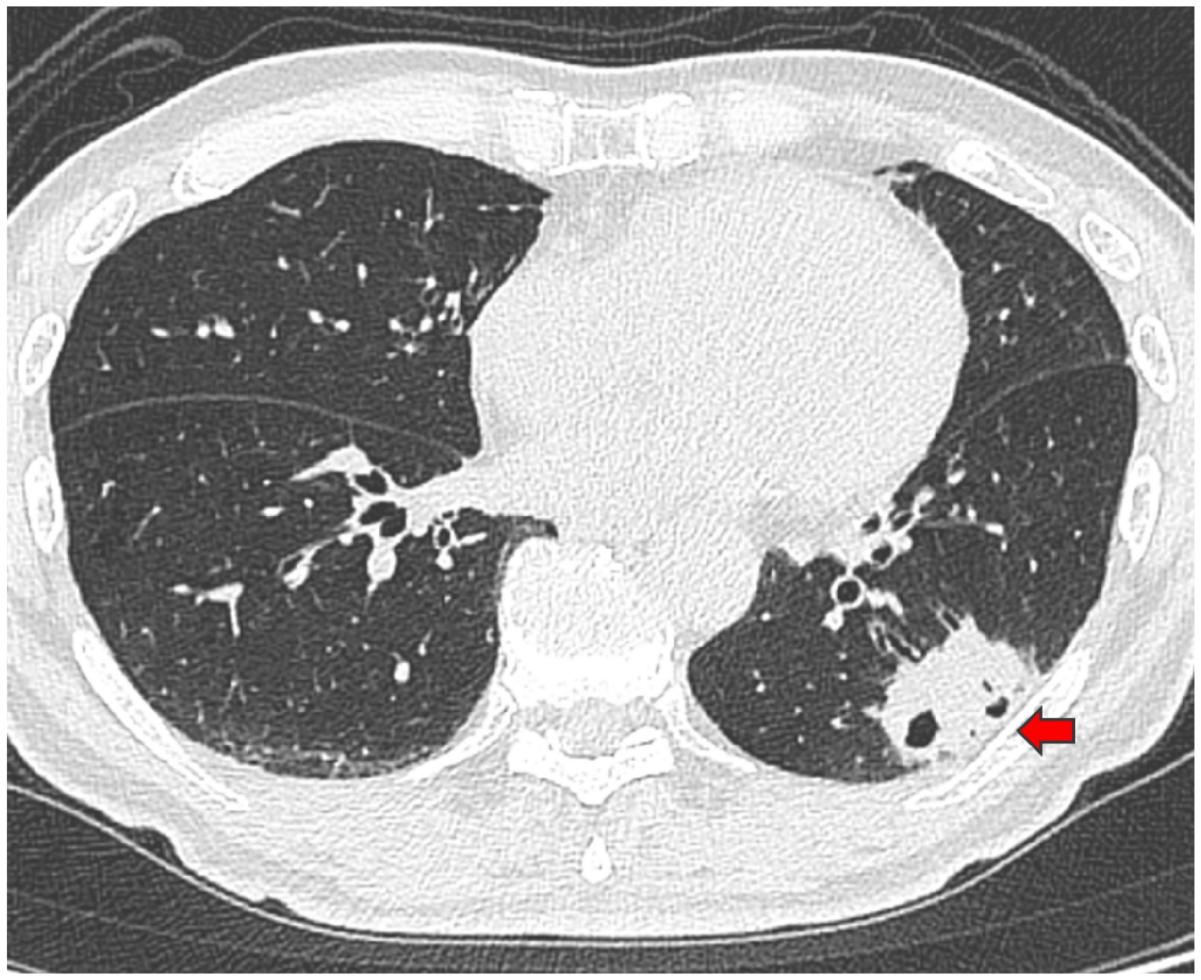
CT findings at the initial and last visits for Case 2. A 41 × 24 mm mass with a cavity was found in the left lower lobe. The arrow shows the mass with a cavity.
